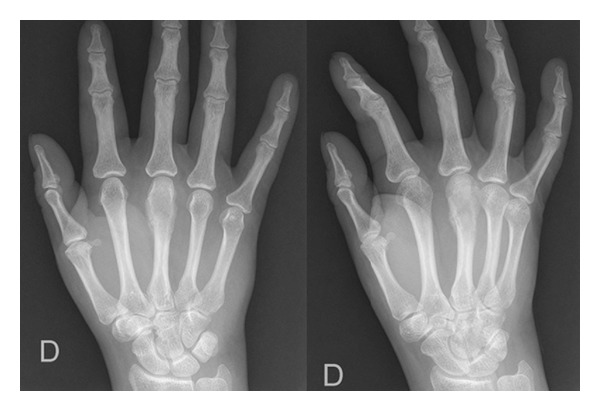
Radiograph taken 3 months postoperatively indicating that the patient had local recurrence.
